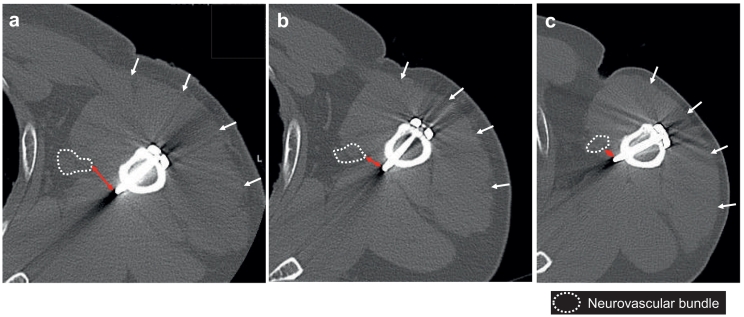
Postoperative computed tomography images of the shoulder at the level of the 2nd distal locking screw. a: One week after surgery of proximal humeral fracture. b: Three months after surgery. c: Three years after surgery. white arrow: edge of the deltoid muscle. Red arrow: distance between the neurovascular bundle and the tip of the screw. (For interpretation of the references to colour in this figure legend, the reader is referred to the web version of this article.)
